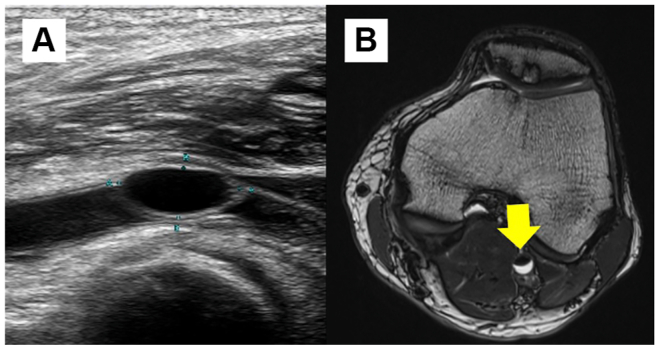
Preoperative imaging of the popliteal artery adventitial cystic disease (ACD). (A) Duplex ultrasound image showing a cystic lesion compressing the arterial lumen. (B) Magnetic resonance imaging demonstrating a high T2-weighted and low T1-weighted signal cyst surrounding the popliteal artery (*arrow*).
